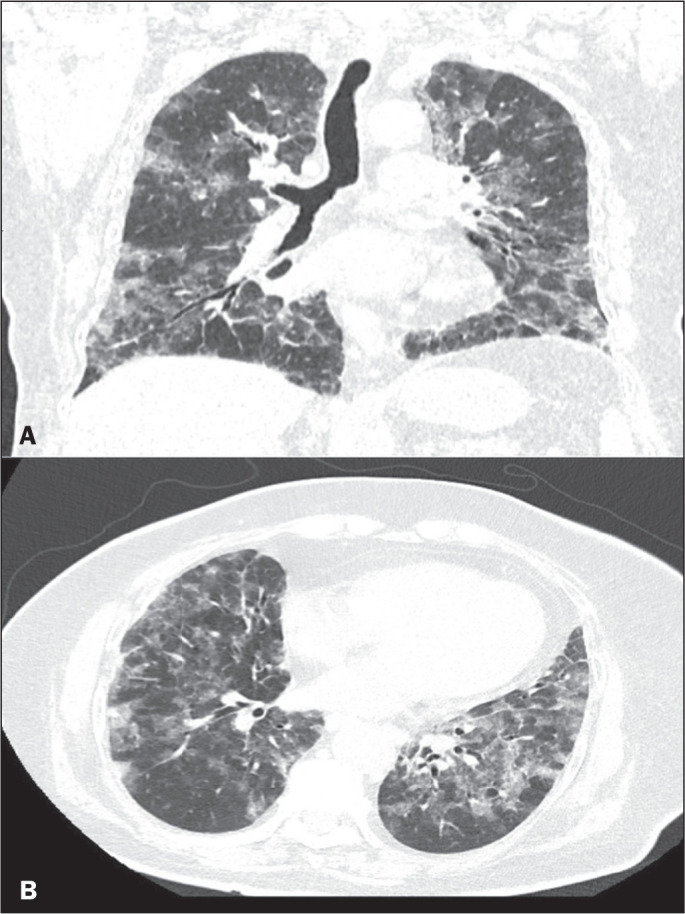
CT findings classified as severe in a 65-year-old unvaccinated female
